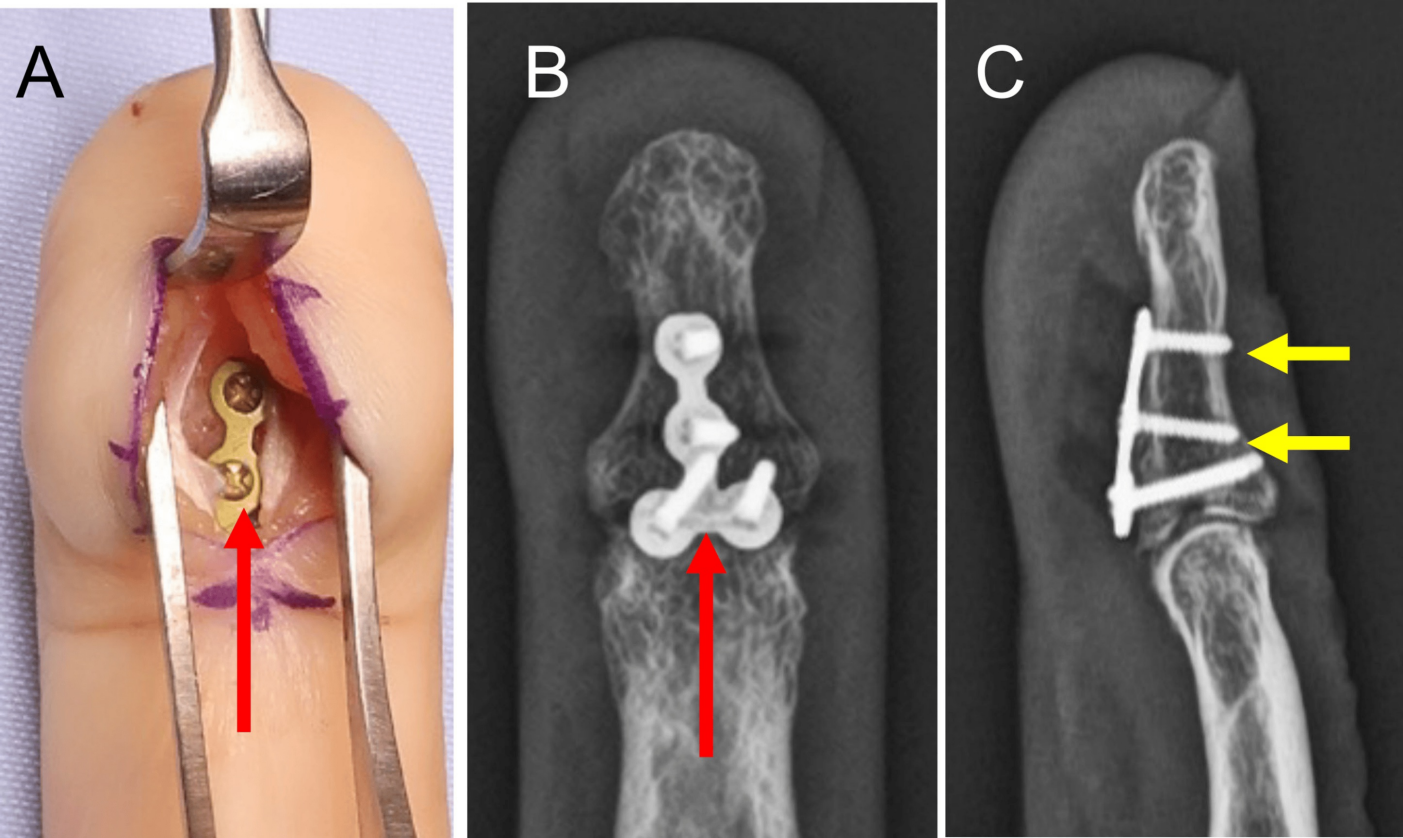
Intraoperative photograph and postoperative radiographs of Patient 1 A T-plate (red arrows) was applied at the volar surface of the distal phalanx (A). The radiographs, in both AP (B) and lateral (C) views, demonstrate the positioning of the plate and reduction of the dorsal subluxation of the DIP joint. The tip of the screws penetrates the dorsal cortex (yellow arrows), and the plate holds the bone fragment in place. DIP: distal interphalangeal
